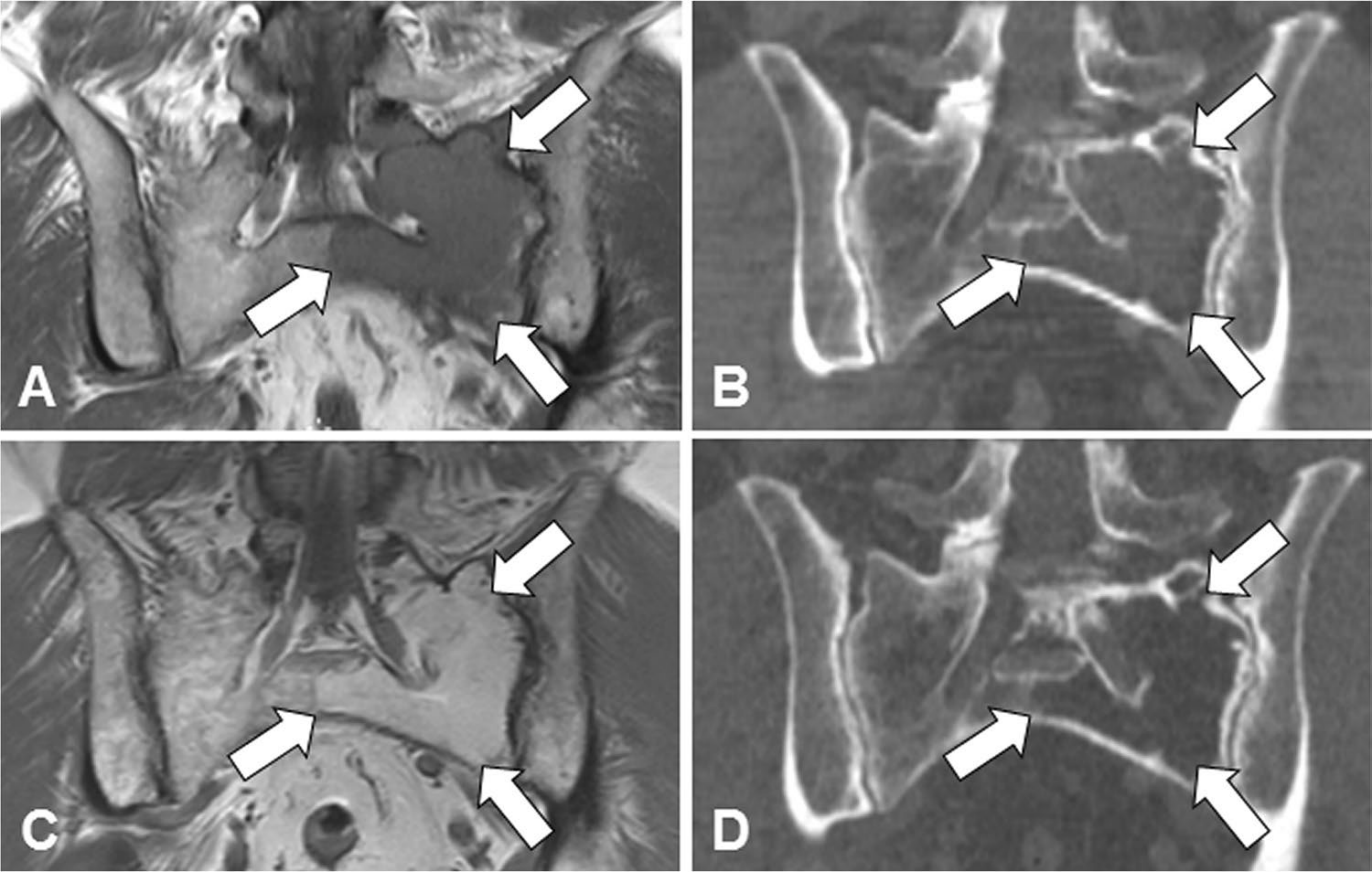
58 year-old man with ND MM (IgG kappa, stage IIIA, ISS 1) treated with high dose cytotoxic treatment and ASCT: value of morphological MRI and limited value of CT for response assessment. A Coronal T1-weigthed image of the sacrum shows extensive bone marrow lesion with low signal intensity within the left portion of the sacrum (arrows). B Corresponding reformatted coronal CT slice shows lytic lesion within the left portion of the sacrum (arrows). C 6-month follow-up coronal T1-weighted image shows disappearance of the left sacral lesion, with complete fatty replacement (arrows); no residual disease was found in the axial skeleton. D 6-month follow-up CT shows no significant change compared to the baseline: persistence of a large lytic lesion within the left sacrum (arrows).
